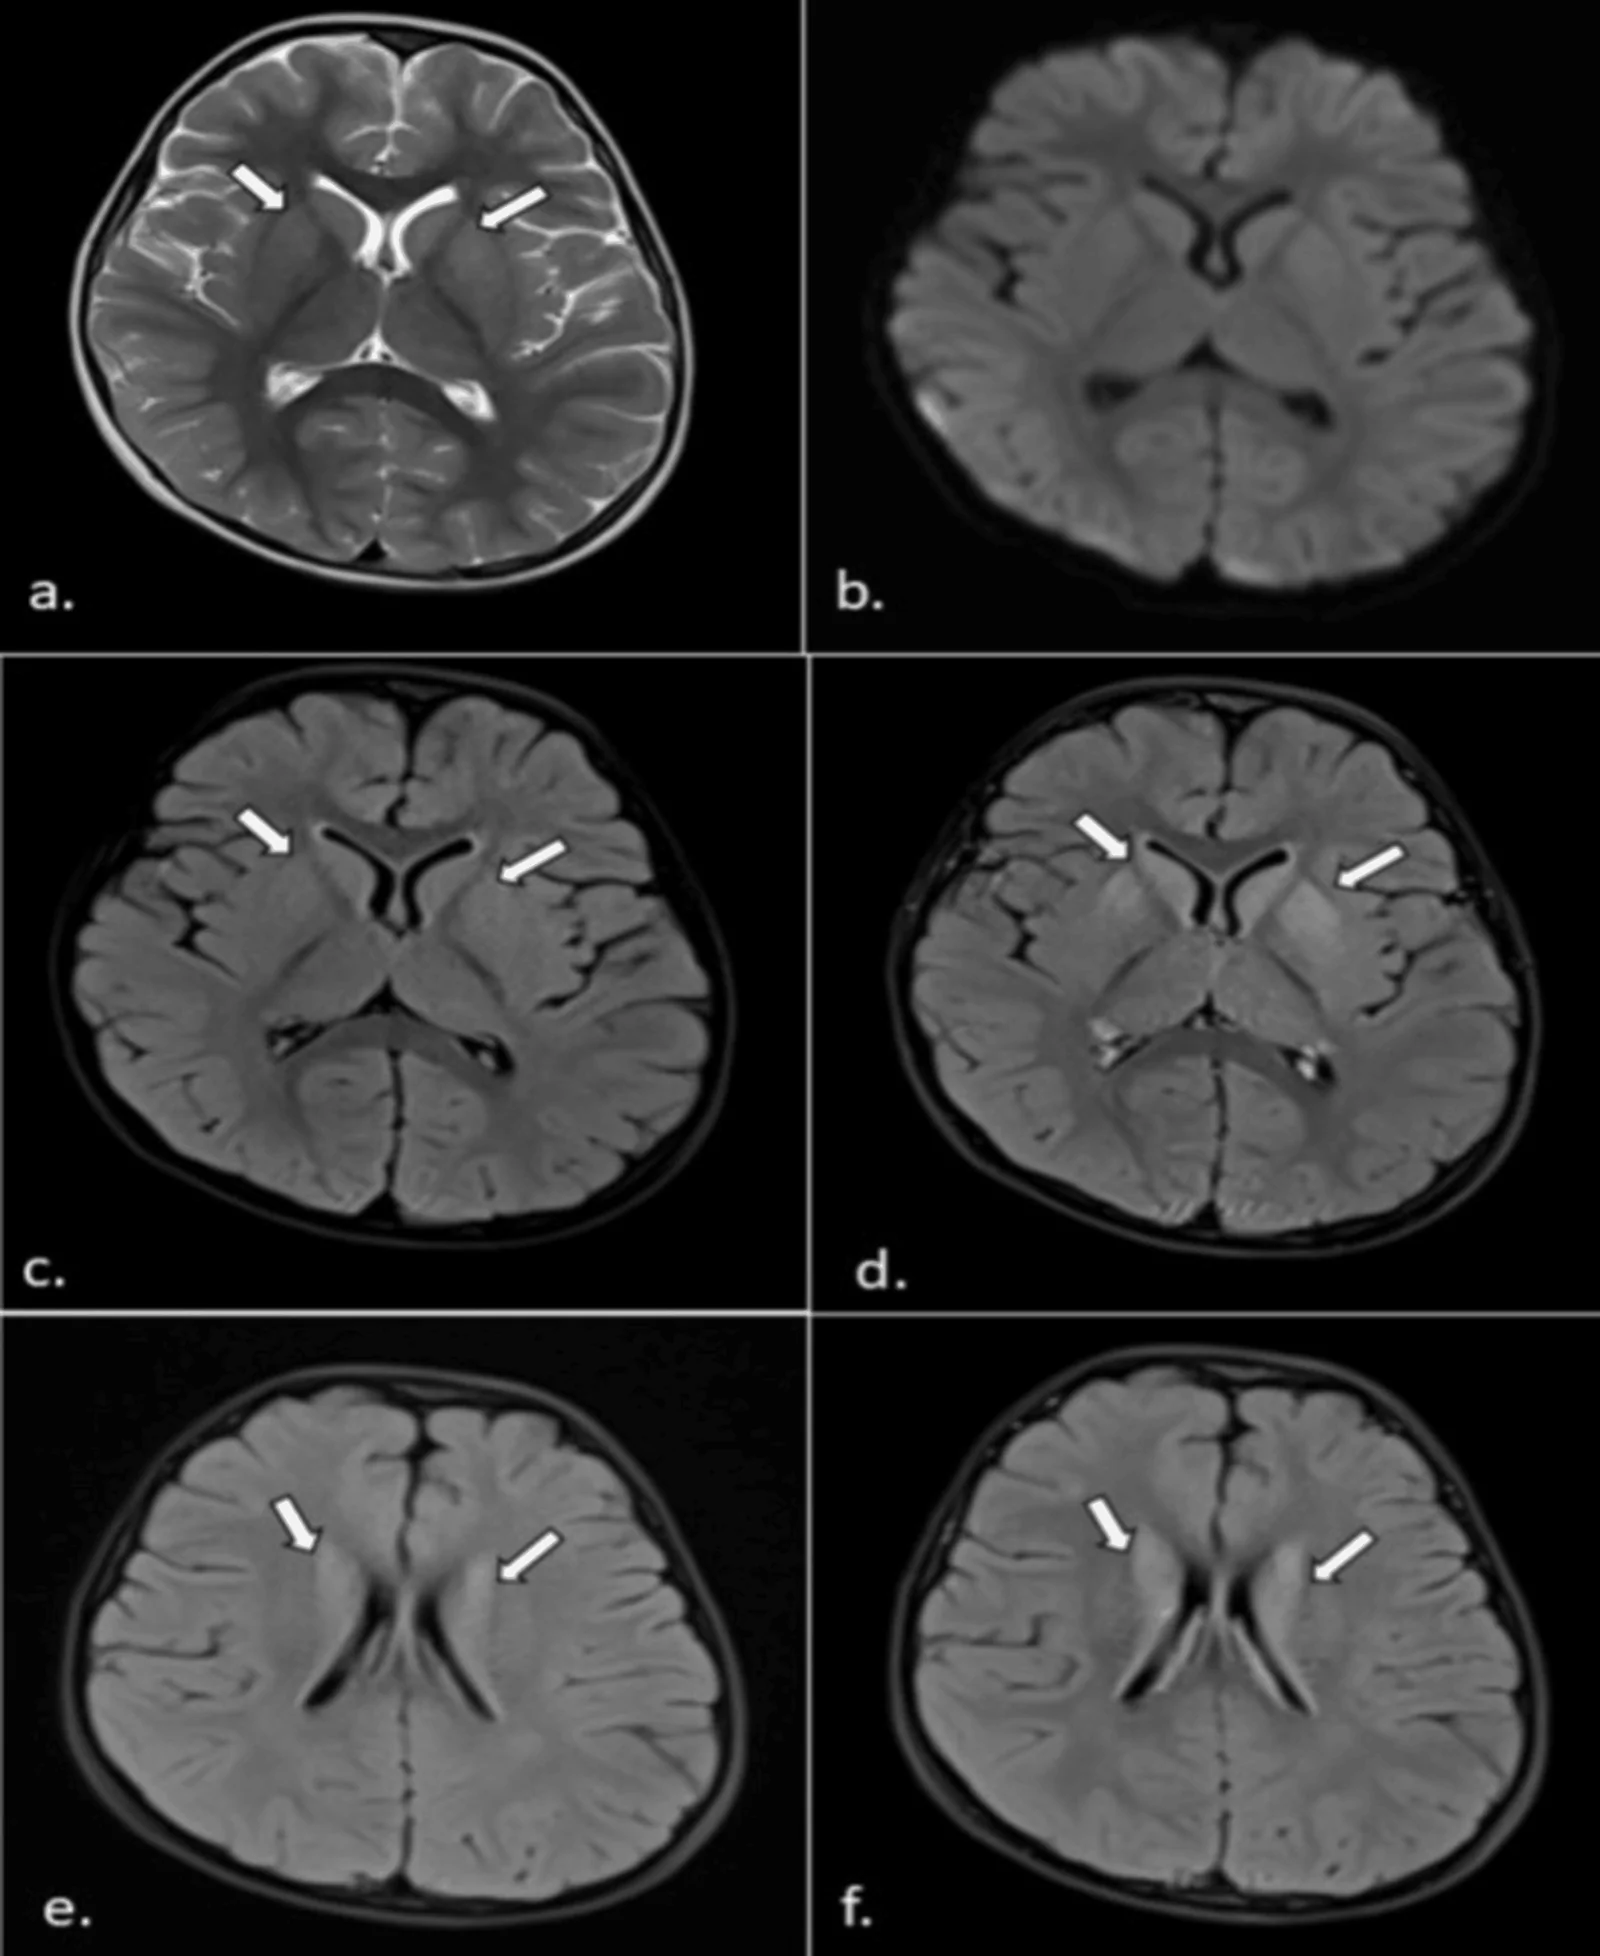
Magnetic resonance imaging of the brain. Axial T2-weighted (a), diffusion-weighted imaging (b), pre-contrast fluid-attenuated inversion recovery (FLAIR) (c), and post-contrast FLAIR (d) image at the level of the basal ganglia showing hyperintensity involving the heads of the bilateral caudate nuclei and bilateral lentiform nuclei (a, c) with no diffusion restriction (b) and showing post-contrast enhancement (d) (arrows). Pre-contrast (e) and post-contrast (f) axial FLAIR image at the level of the caudate nucleus showing hyperintensity involving the bilateral caudate nuclei with enhancement (arrows).
